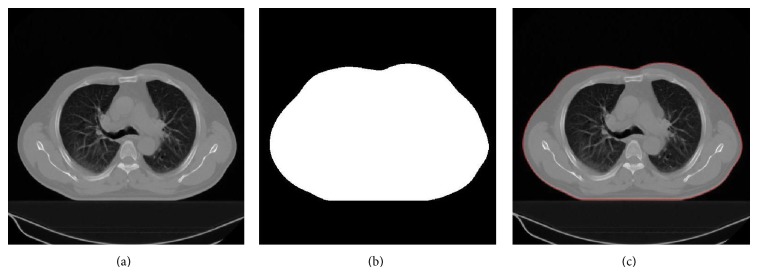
Segmentation of the body region: (a) original CT slice, (b) segmented body region in white, and (c) body contour in red superimposed on the original slice.
